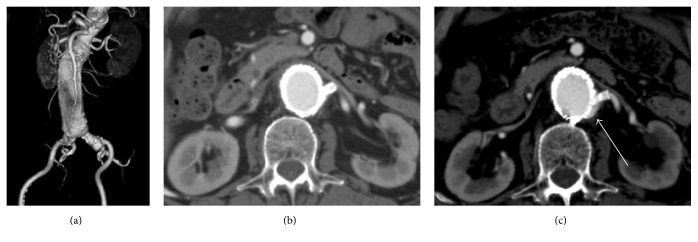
(a) Preoperative 3-dimensional computed tomography with the left anterior oblique view showed a juxtarenal aneurysm measuring 59 mm in diameter with a short proximal neck. (b) Early postoperative computed tomography showed a patent endograft and bare stent to the left renal artery without any endoleaks. (c) Two years after endovascular aneurysm repair, computed tomography showed the enlargement of the aneurysmal sac with a type Ia endoleak (white arrow).
